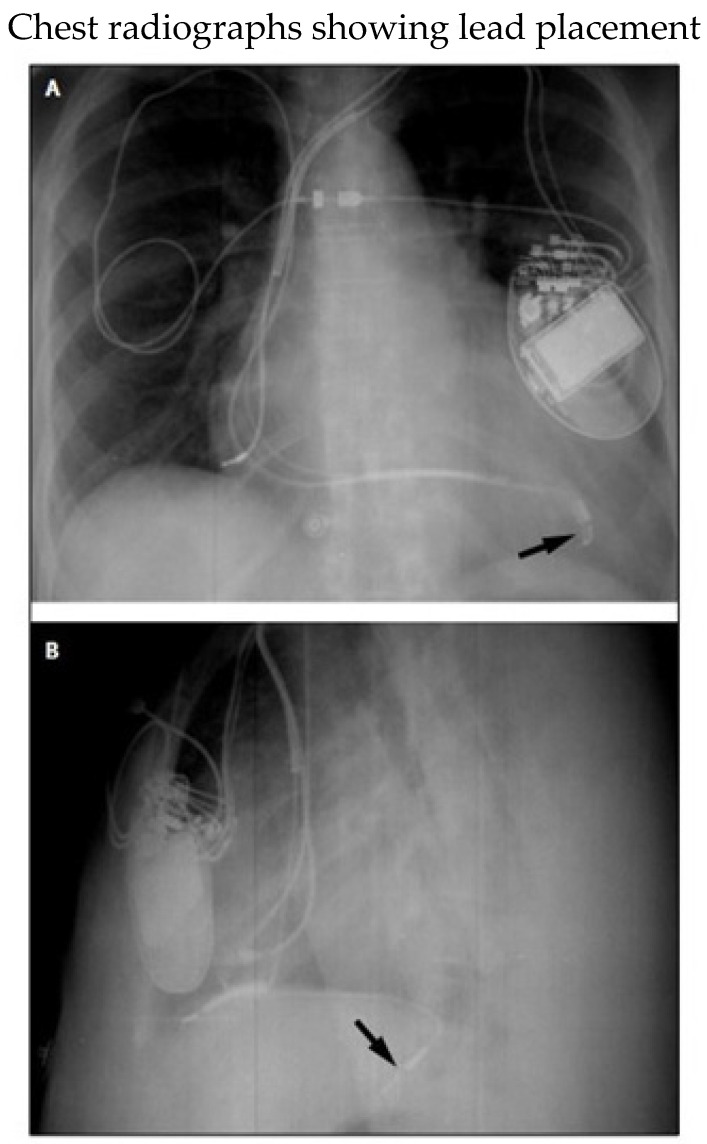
(A) Posteroanterior and (B) lateral radiographs showing tip of left ventricular lead (arrows) in a tributary of the middle cardiac vein. Despite proximity to the left hemidiaphragm, phrenic near stimulation did not take place. The right ventricular lead points anteriorly toward the rib cage. Reproduced from reference [78] with permission.
